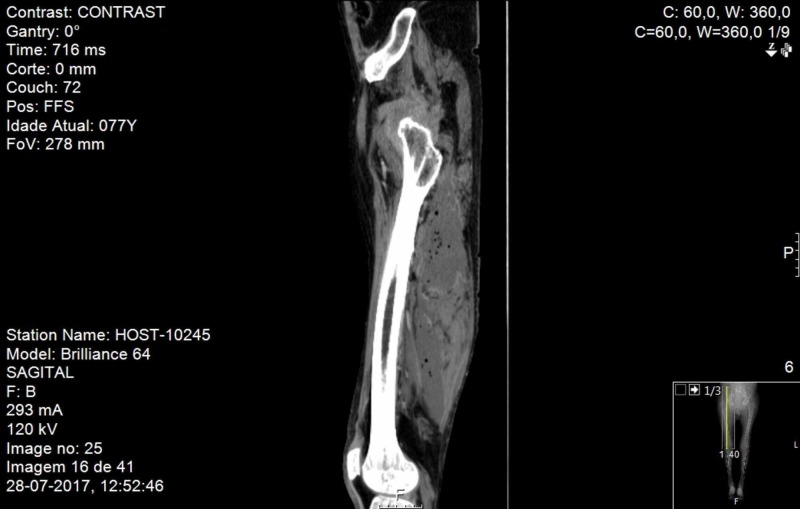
Right lower limb CT scan, sagittal plane Right lower limb computerized tomography scan, sagittal plane, revealing a gas collection in the region of the right iliacus muscle
